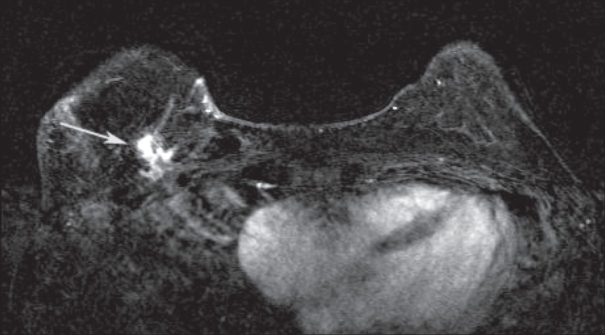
Axial subtracted DCE-MRI image of a patient with right TRAM flap reconstruction after mastectomy. This patient presented with a new thickening situated medially in the reconstructed right breast. DCE-MRI demonstrated a spiculated mass (arrow) with abnormal enhancement. The patient turned out to have widely metastatic disease at the time. She refused further evaluation or treatment
